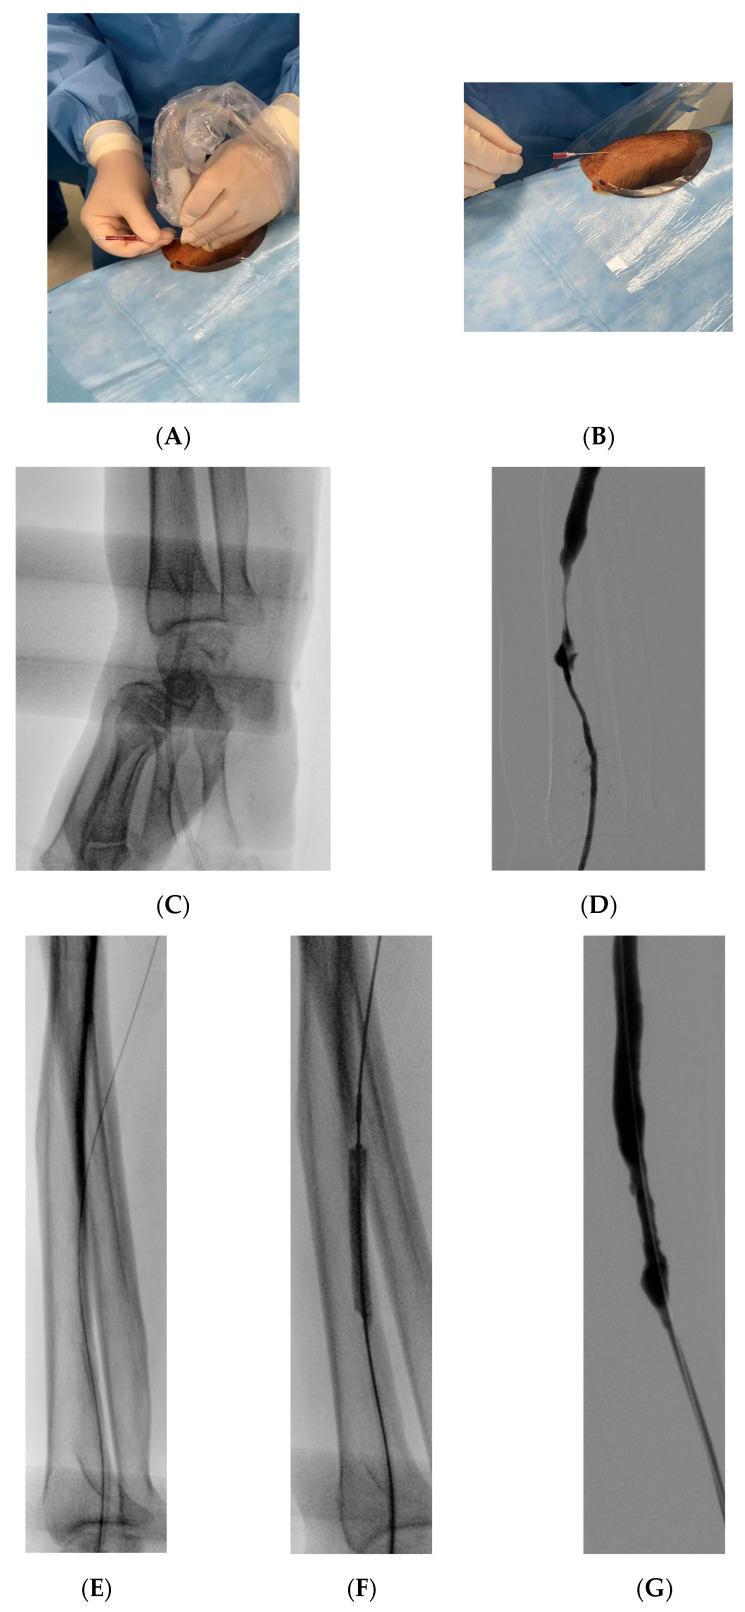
The patient’s forearm is positioned in a relaxed pronated position and a gauze roll is placed under the wrist to keep the hand flexed, thus exposing the anatomical snuff box. Arterial puncture of the distal radial artery is performed under ultrasound guidance with a 21-gauge micro-puncture needle, and a 0.014” guidewire is retrogradely advanced in the radial artery (A,B). Fluoroscopic check confirming the correct positioning of a 4 Fr introducer sheath through the distal radial artery (C). The angiogram revealed a significant stenosis in the mid-to-distal segment of the radial artery, just proximal to the radiocephalic dialysis fistula. Notably, the positioning of the introducer sheath in the distal radial artery provided sufficient room to fully advance the introducer into the vessel, ensuring its secure placement (D). The radial artery stenosis was successfully crossed with a 0.014” guidewire and treated with angioplasty using a 4 mm balloon, achieving an excellent final angiographic outcome (E–G).
